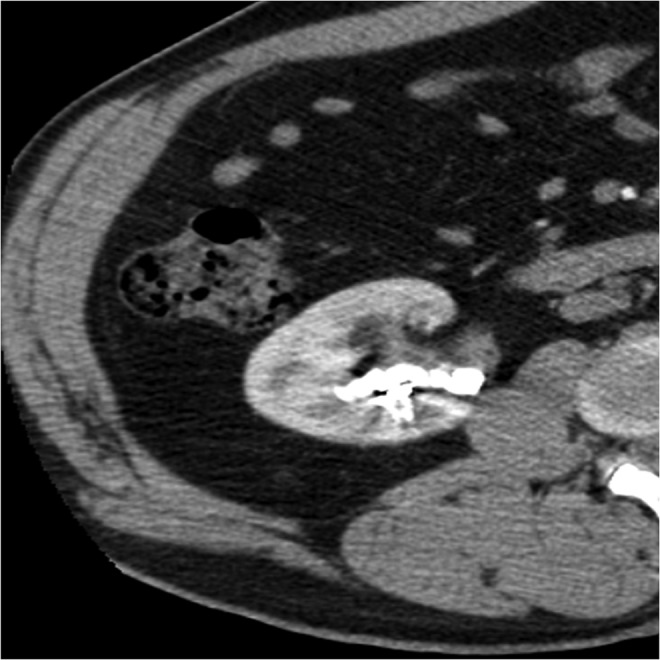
Preoperative CT scan showing metal coils of previous SRAE. SRAE, selective renal artery embolization.
